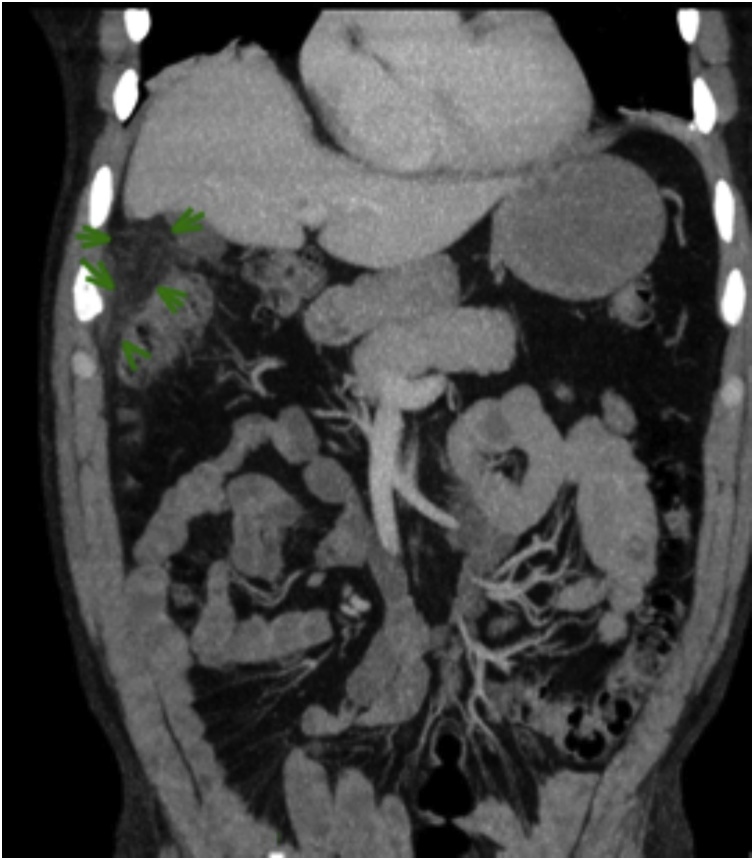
CT abdomen showing a fat density structure, approximately 61 × 25 × 25 mm, abutting the fundus of the gallbladder and the adjacent liver and is in keeping with epiploic appendagitis).
